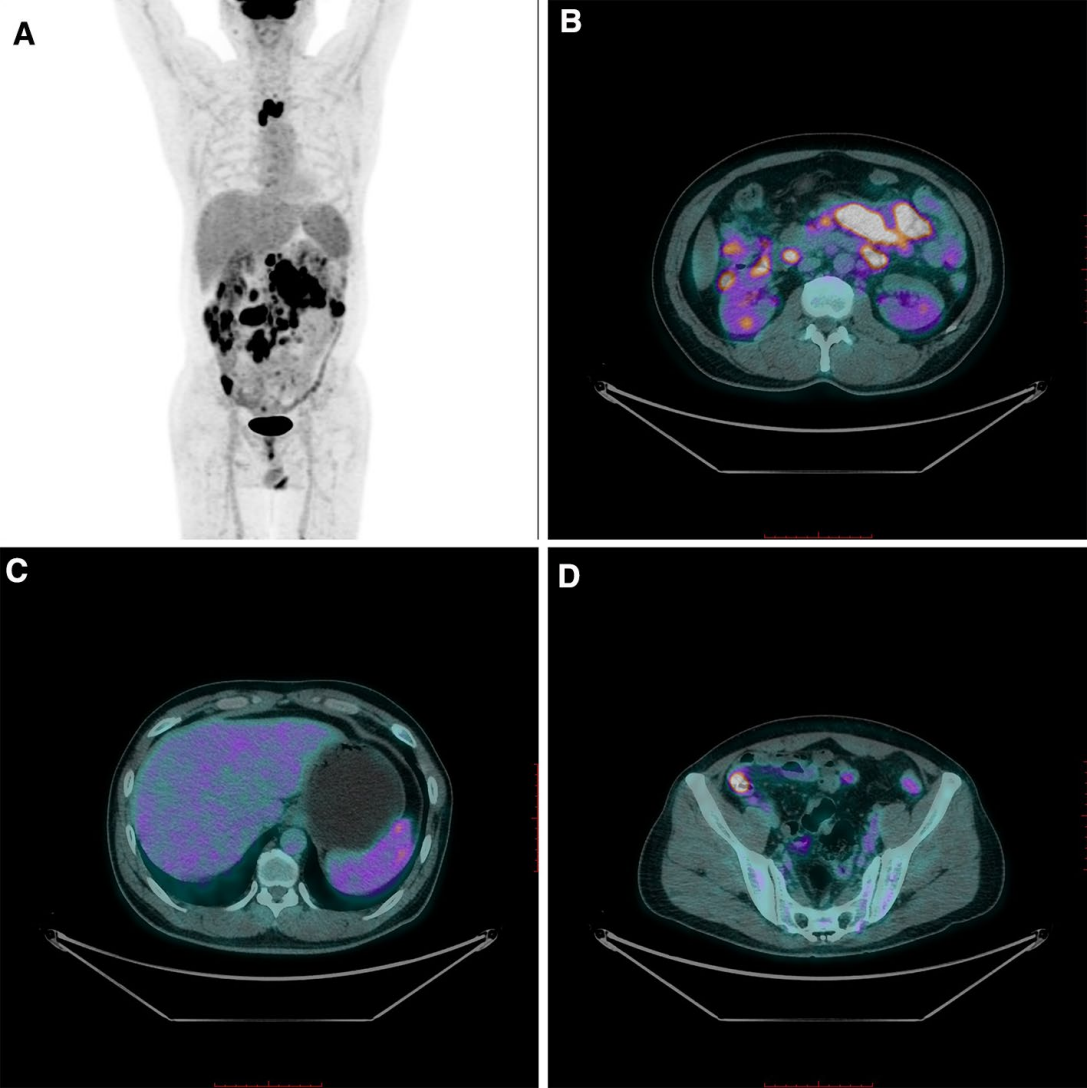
PET-CT examination revealed multiple enlarged lymph nodes with abnormally increased FDG metabolism (A, B). Diffuse increase in FDG metabolism in the spleen (C). Multiple patches of increased FDG metabolism in the ascending and descending colon (D)
